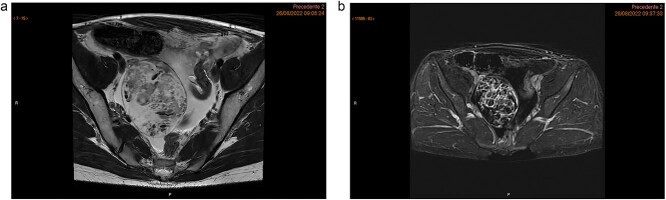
Pelvic MRI highlighting a heterogeneous right ovarian lesion with high signal on axial T2 WI (a), and irregular enhanced septum on DCE T1 WI (arrow). Ascites and a pelvic nodule with fat and contrast enhancement (arrow head) (b).
